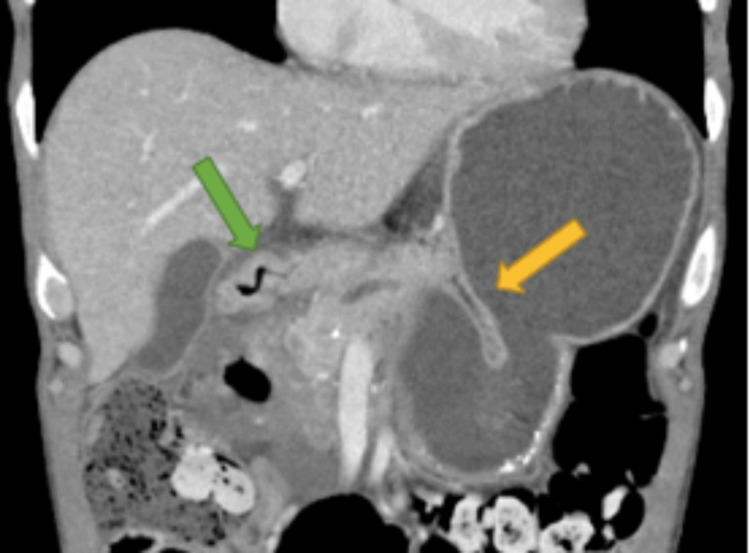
CT abdomen and pelvis with IV contrast showing severe stenosis at the second portion of the duodenum with ulcer (green arrow), and a gastric closed loop obstruction (yellow arrow)
